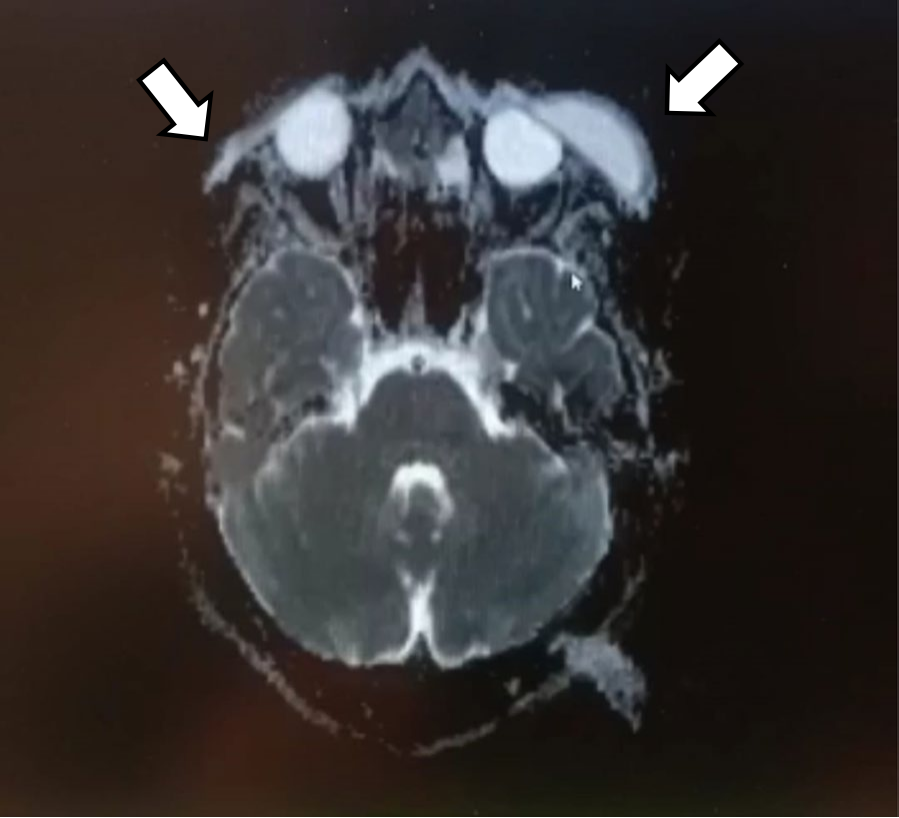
Magnetic resonance imaging brain T2 image view showing enlargement if the bilateral lacrimal glands (arrows), with a left sided collection at the site left lacrimal gland (left-sided arrow)
